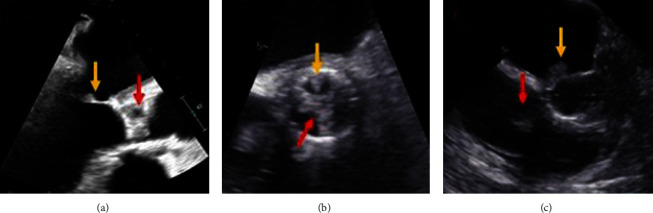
Transesophageal echocardiography of infected TAVR. (a) Thickened TAVR leaflets (red arrow) and vegetation of the mitral valve (yellow arrow). (b) Thickened TAVR leaflets (red arrow) and vegetation of the aortic cusp (yellow arrow). (c) Vegetations of the tricuspid valve (red arrow) and aortomitral continuity (yellow arrow).
